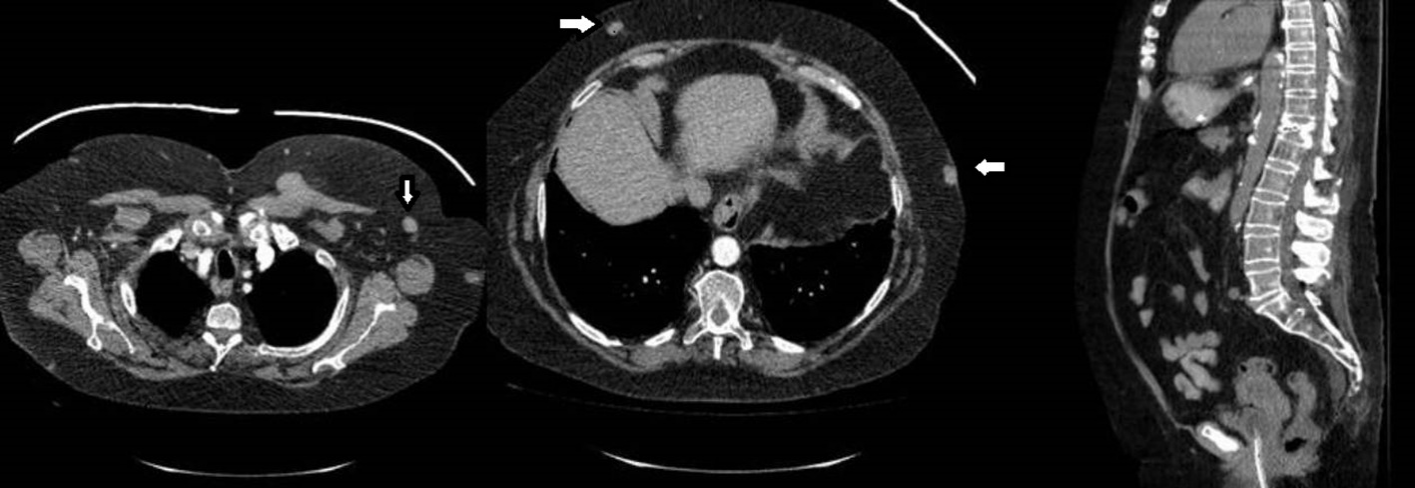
CT-TAP shows multiple heterogeneous soft tissue nodules in neck, chest wall, axilla, anterior abdomen with hilar and mediastinal lymphadenopathy.
